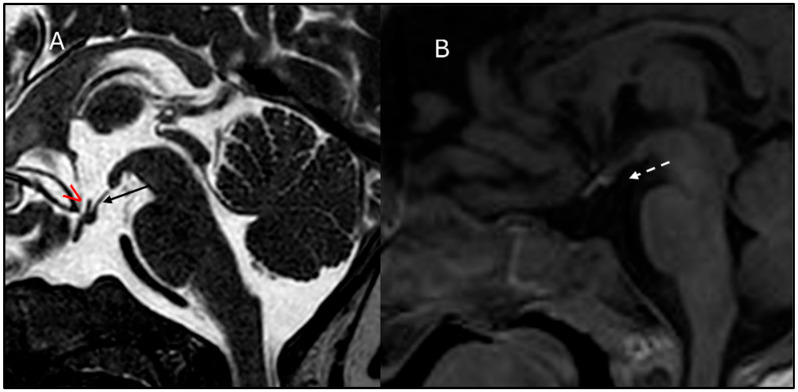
Family B, III.4 Brain MRI. Sagittal MR images T2 weighted (A) and T1 weighted (B) Pituitary morphology: Hypoplastic anterior pituitary lobe (>), thin pituitary stalk (arrow), and ectopic posterior lobe located at the level of the median eminence (dashed arrow).
